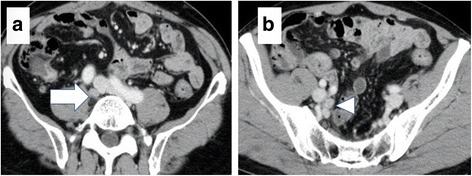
Contrast-enhanced computed tomography. Images show swollen lymph nodes a in the right common iliac artery area (*arrow*) and b right internal iliac artery area (*arrowhead*)
